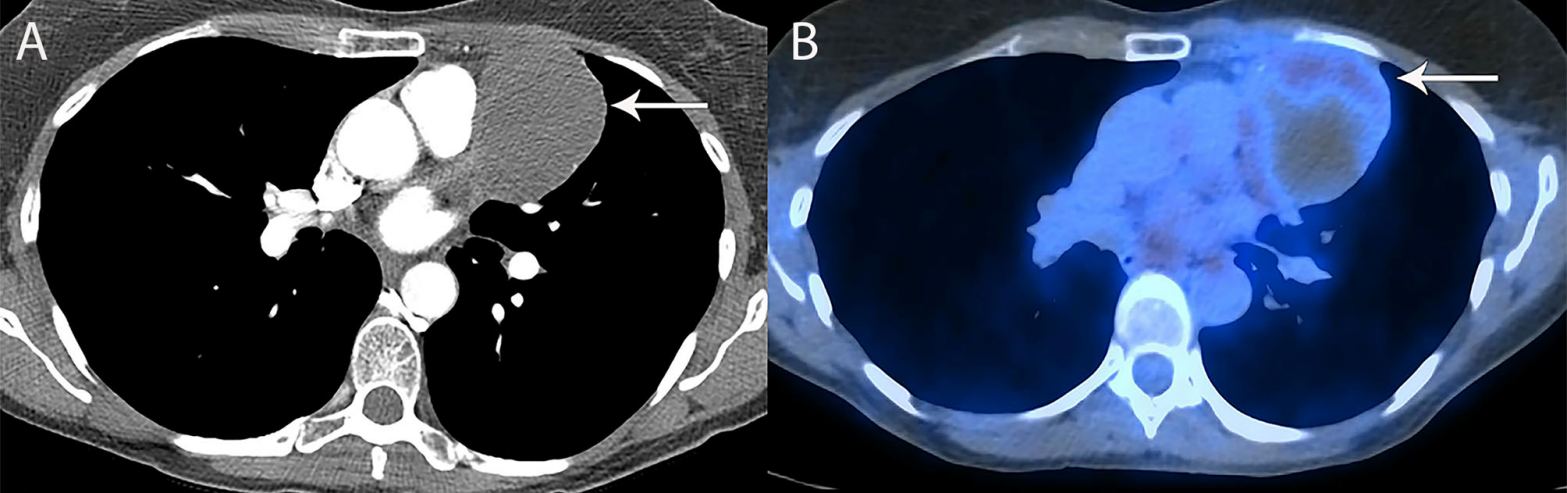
53 year old woman with thymoma. (A) CT shows the left prevascular mediastinal mass (arrow) is mostly homogenous, with low attenuation measuring 10 Hounsfield Units suggesting a cystic component, yet has a denser component of 48 Hounsfield Units along the anterior peripheral aspect suggesting some solid component. (B) PET/CT shows FDG uptake with SUVmax of 3.4 along the anterior peripheral aspect of the mass (arrow). At resection, pathology showed thymoma with cystic component and no invasion of the pericardium or lung.
